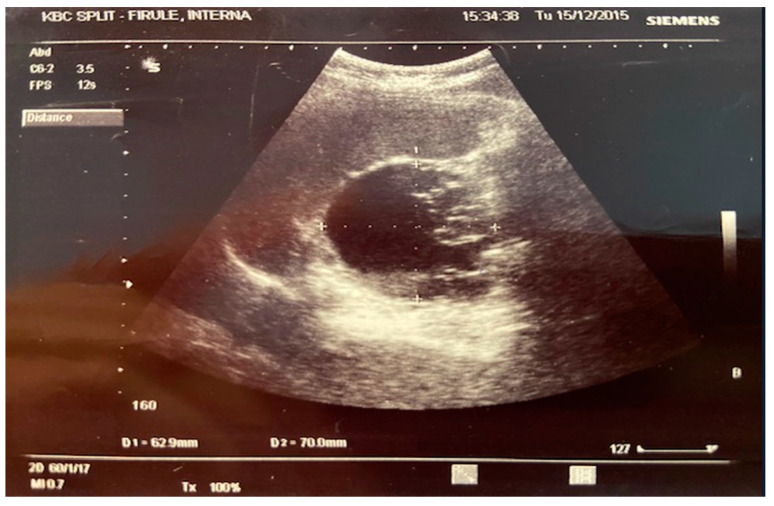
Ultrasound scan of the abdomen with findings of a cyst in the region of the pancreatic trunk.
